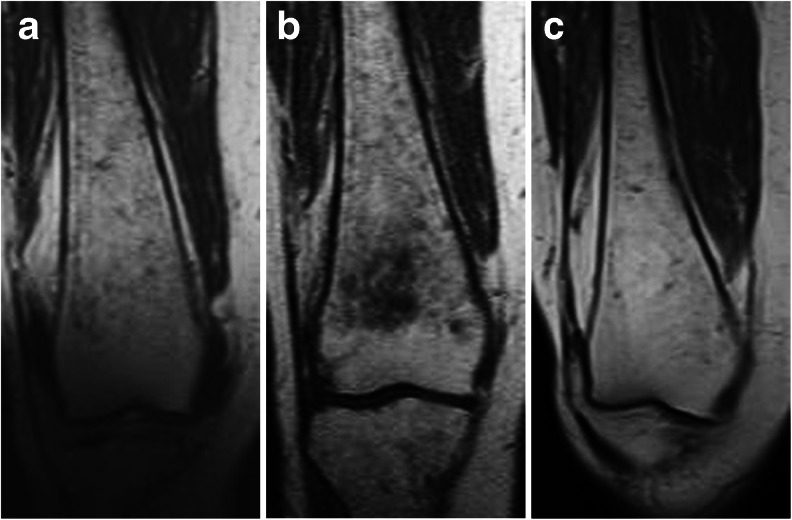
Resolution of indeterminate lesion and continued dark marrow improvement in one patient. Coronal T1-weighted MR images of the distal right femur of a 55-year-old female patient at 36 months (a) and 48 months (b) demonstrate an interval increase in dark marrow in the distal femoral diaphysis and metaphysis with a new indeterminate metaphyseal bone lesion at 48 months. At 60 months (c), the bone lesion resolved and dark marrow continued to improve, even as compared to 36 months, with near-complete resolution of dark marrow in the distal femoral diaphysis and metaphysis
